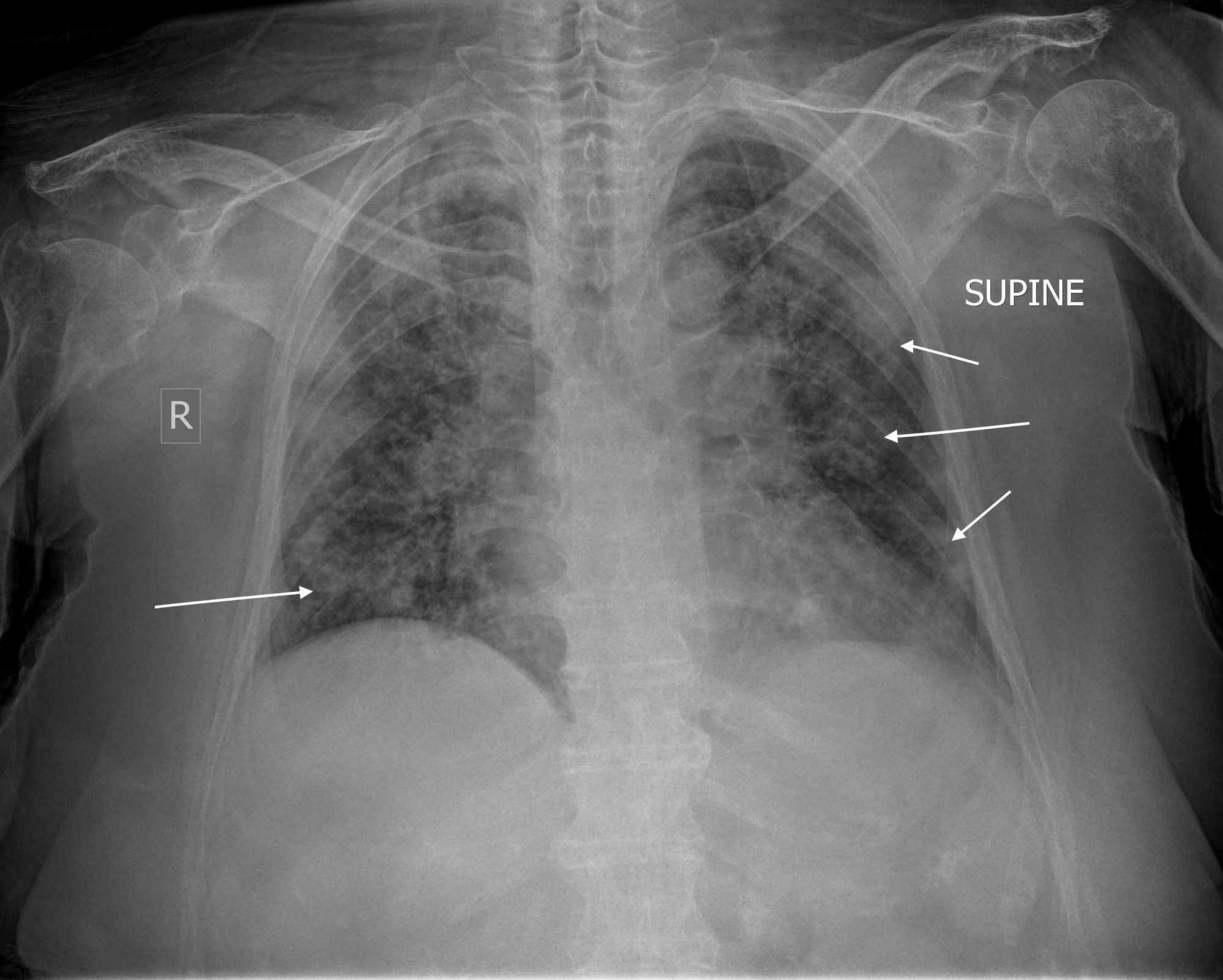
Anteroposterior chest radiograph demonstrating pneumonia of both lungs with typical ground-glass bilateral, multifocal, peripheral opacities (arrows) R: right
